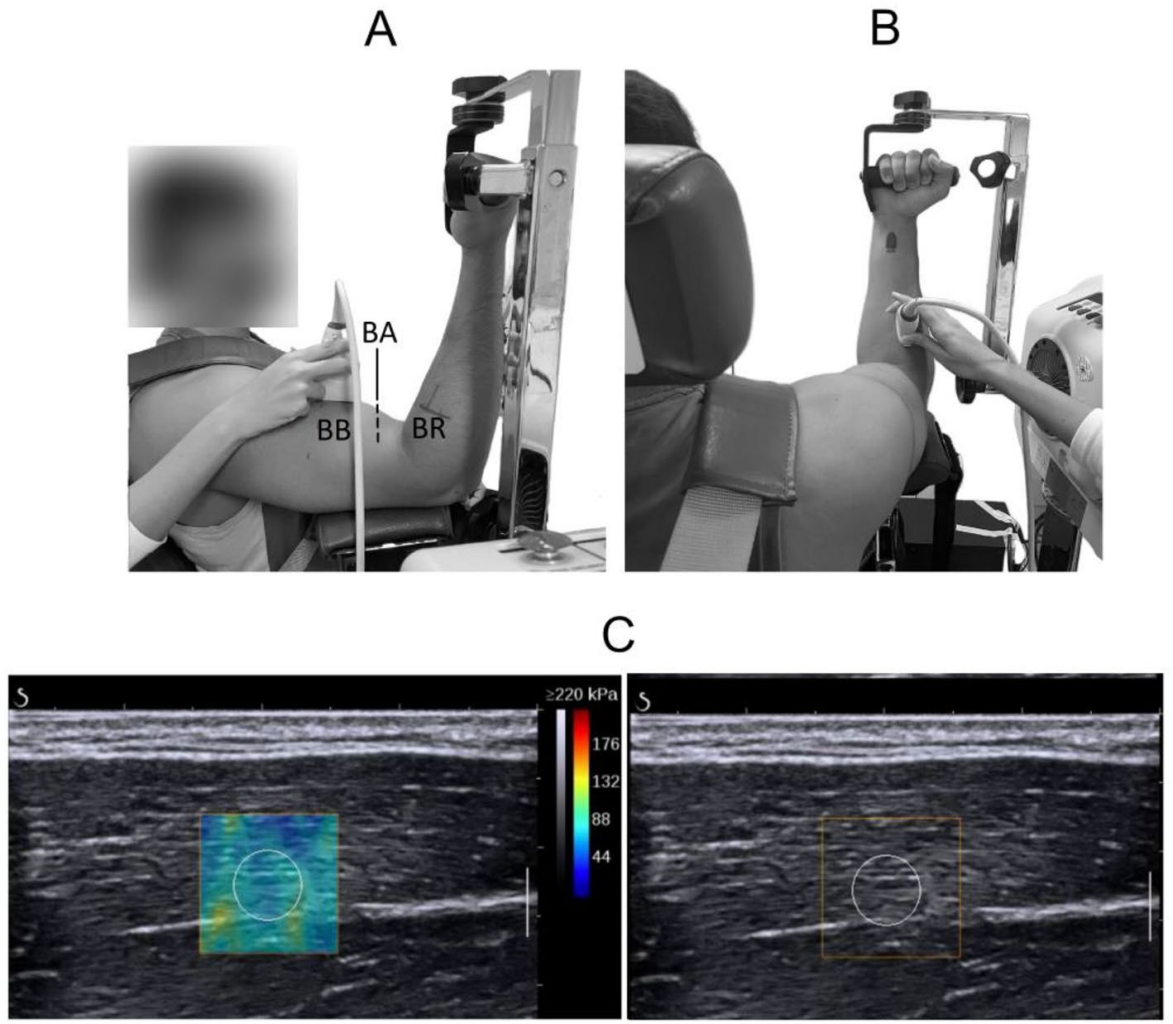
Examples of $${\mu }_{L}$$ measurements. (A,B) Placement of the ultrasound transducer over the free surface of the biceps brachii (BB) and brachioradialis (BR) muscles, respectively. (C) Echographic image showing the ROI chosen for the measurements. At the right, the echographic image without the color map is shown. The colored region in the image at the left depicts the shear elasticity map according to the corresponding color scale.
